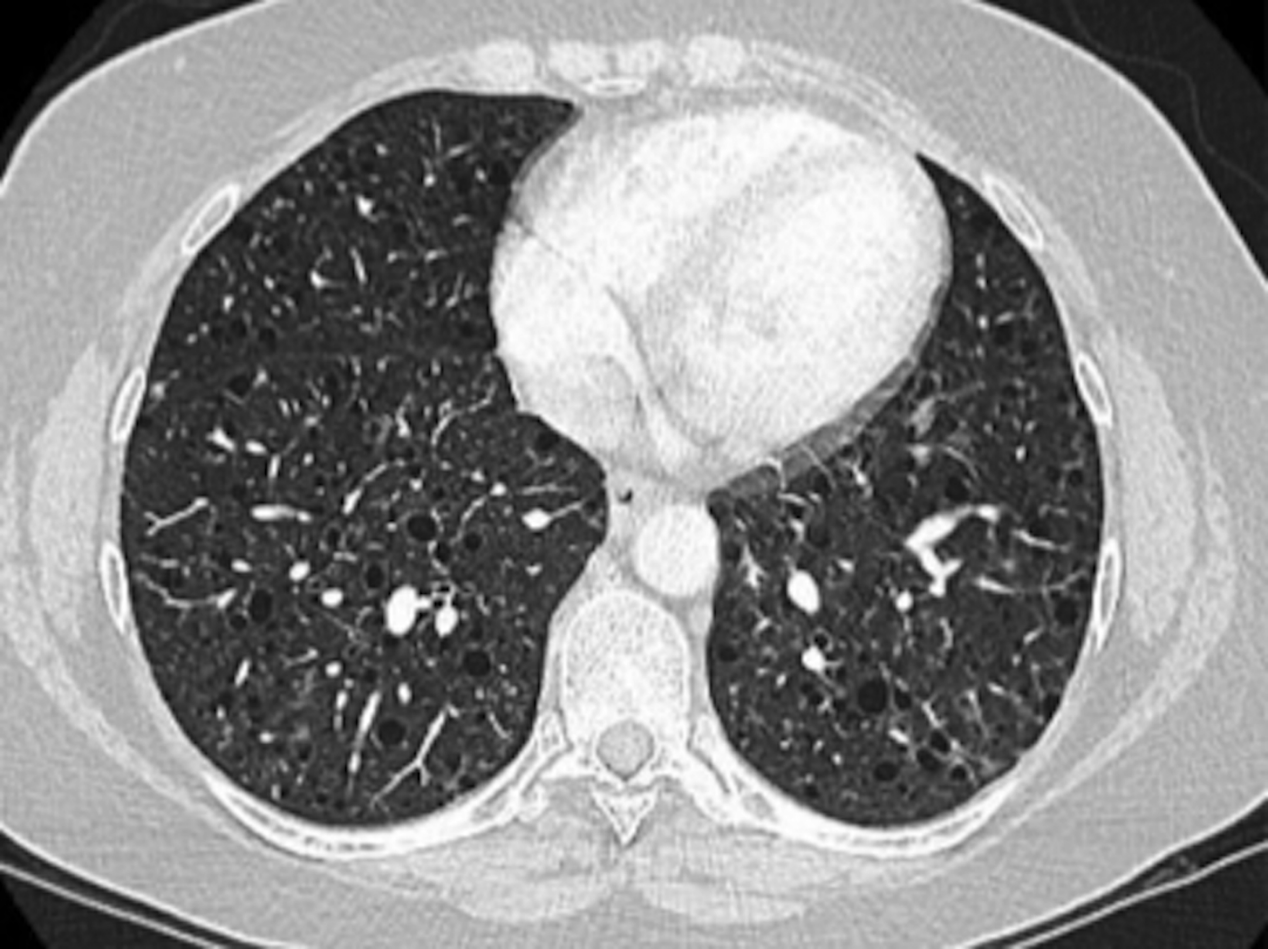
A 45‐year‐old lady presents with acute dyspnoea. Computed tomography (CT) pulmonary angiogram demonstrates multiple bilateral cysts that are evenly distributed throughout the pulmonary parenchyma. Ground‐glass nodules were noted; there were no solid parenchymal nodules or lymphadenopathy.
